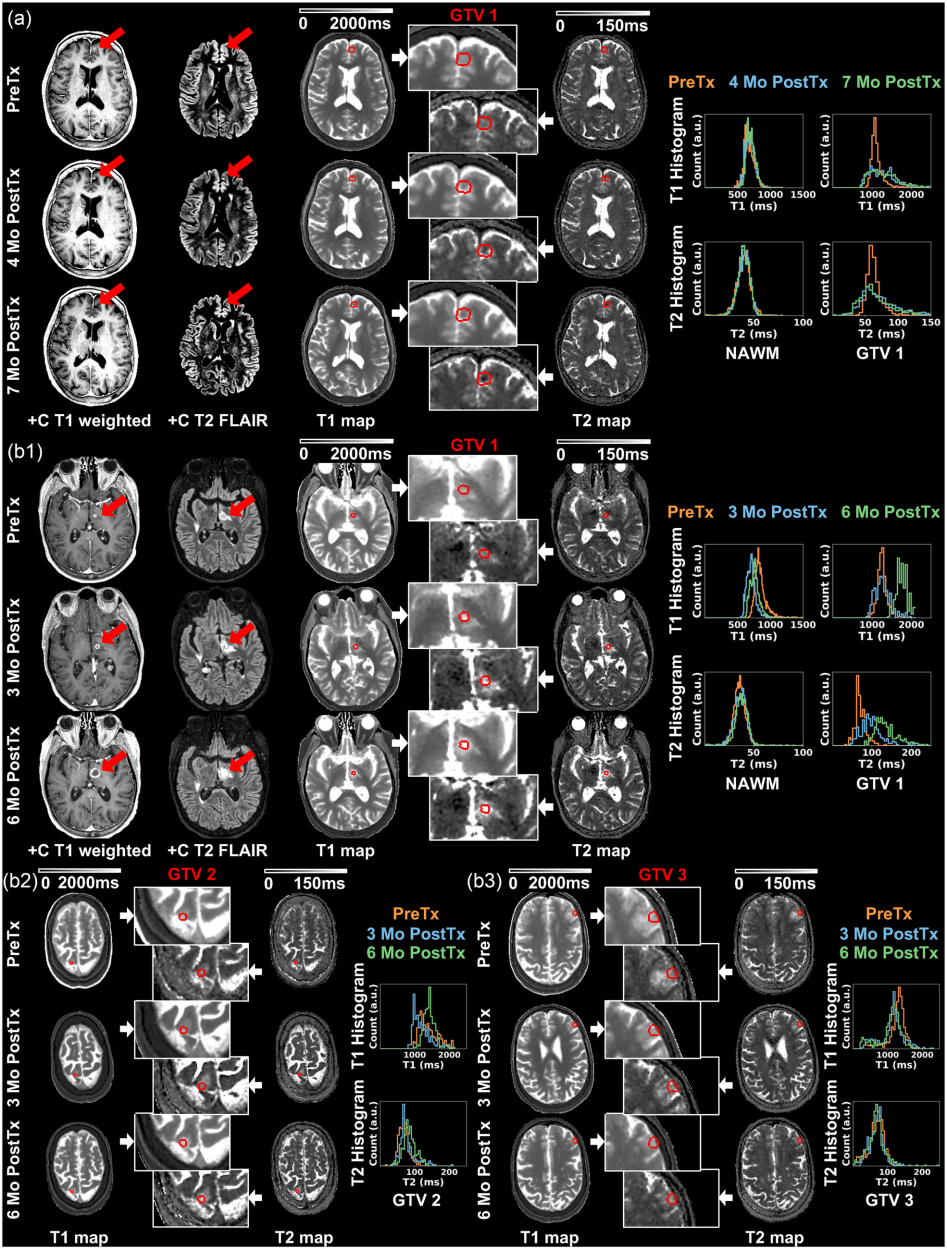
Longitudinal post‐contrast T1 weighted images and T2 FLAIR, DL‐MUPA derived T1 and T2 maps (global and magnified to metastases), and quantitative T1 and T2 histograms of normal appearing white matter (NAWM) and gross tumor volume (GTV), (A) presenting one resolved lesion for brain metastases Patient A and (B1‐3) presenting three necrotic lesions for Patient B.
